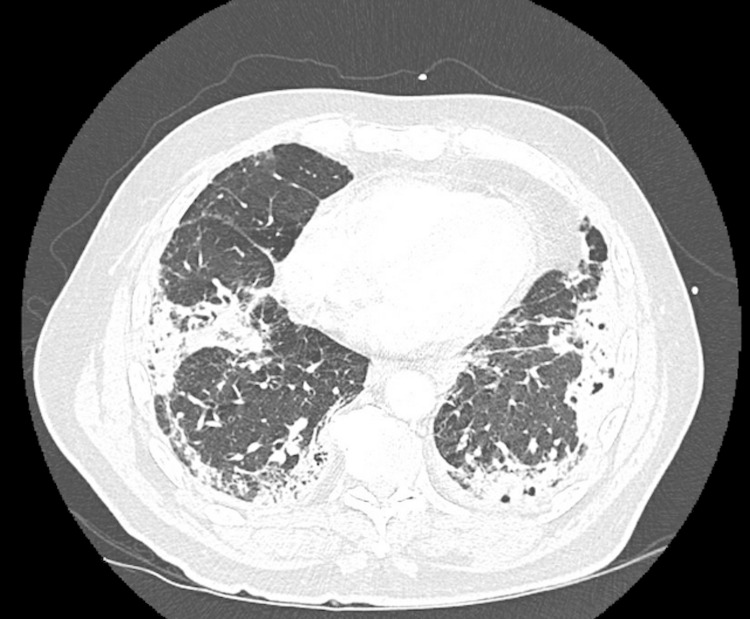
CT chest CT chest showed multiple predominantly peripheral areas of consolidation in both lungs. The consolidation appeared dense with cystic areas within the consolidation, raising concern for necrotizing pneumonia CT: computed tomography
